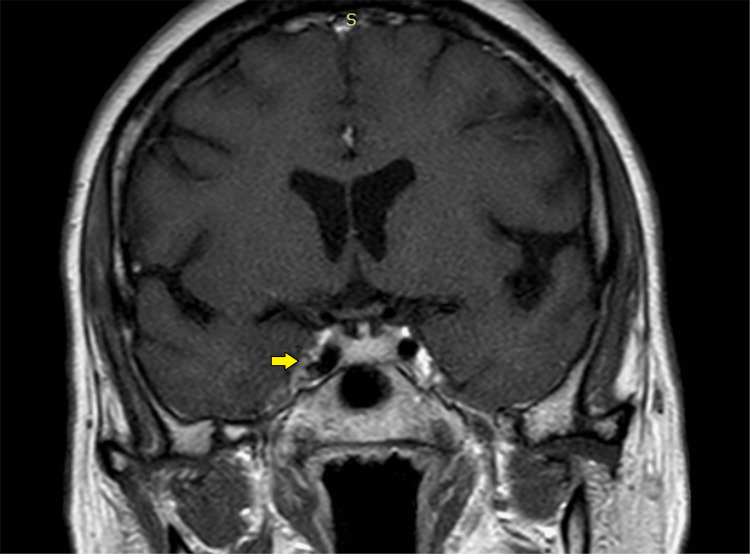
Magnetic resonance imaging (T1 post-contrast coronal section) showing focal involvement of the right cavernous sinus (yellow arrow) by the disease process.
